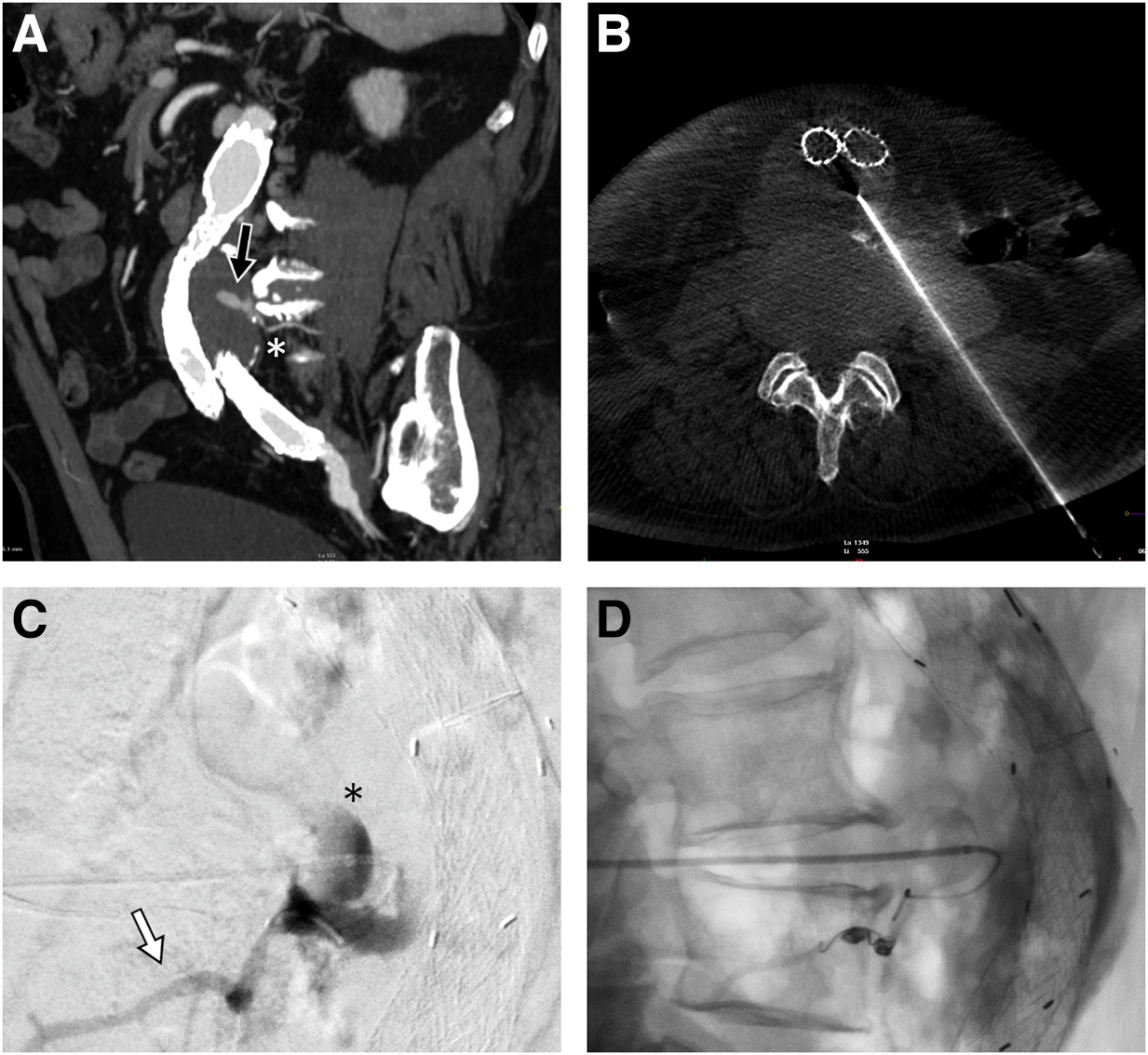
Sagittal MIP of the pre-procedural CTA showing the posterior endoleak (black arrow) along with the lumbar feeder (white asterisk) (A). Cone beam CT confirming the 21G needle tip positioning inside the aneurysmatic sac (B). In lateral projection, the injection from the microcatheter shows the endoleak nidus (black asterisk) and the lumbar feeder (white arrow) (C), which is embolized with detachable microcoils (D).
